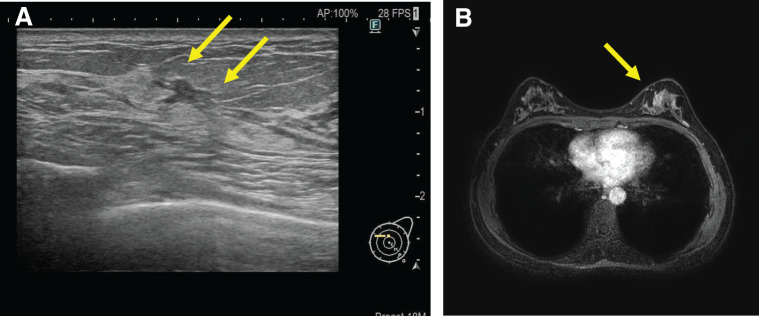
Images of the patient’s preoperative evaluation. (A) Ultrasonography of the left breast showed a 2.0 cm non-mass lesion with intraductal calcification without nipple involvement. (B) MRI showed suspicions of clumped enhancement in the upper-inner quadrant area of the left breast.
